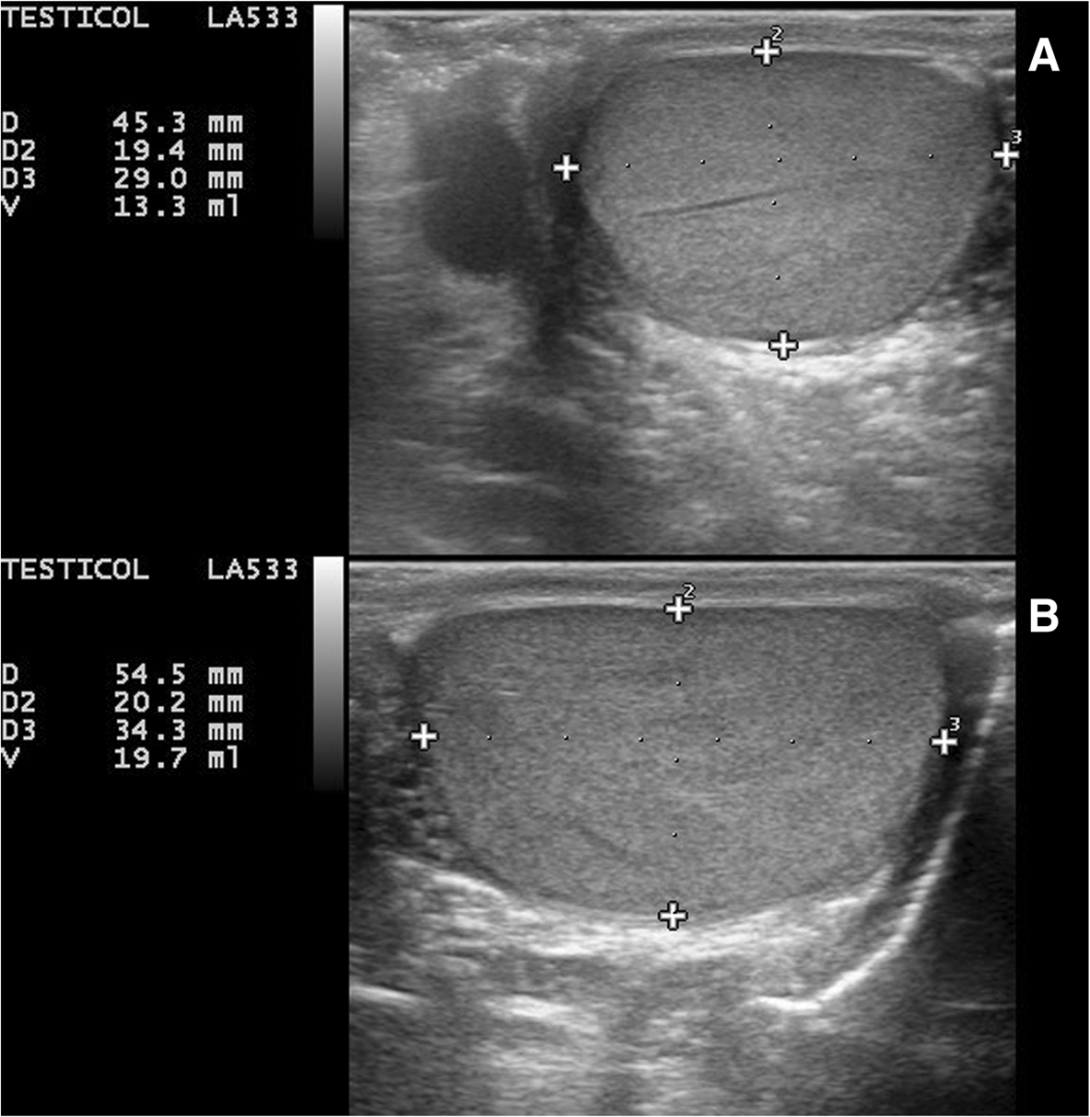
Testicular ultrasound of our 15-years-old patient showed normal sonographic appearance of both testes and epididymides and confirmed volumetric asymmetry (right testis measuring 54.5 x 20.2 x 34.3 mm with an estimated volume of 19.7 ml, left testis measuring 46.2 x 18.6 x 29.1 mm with an estimated volume of 13.3 ml).
